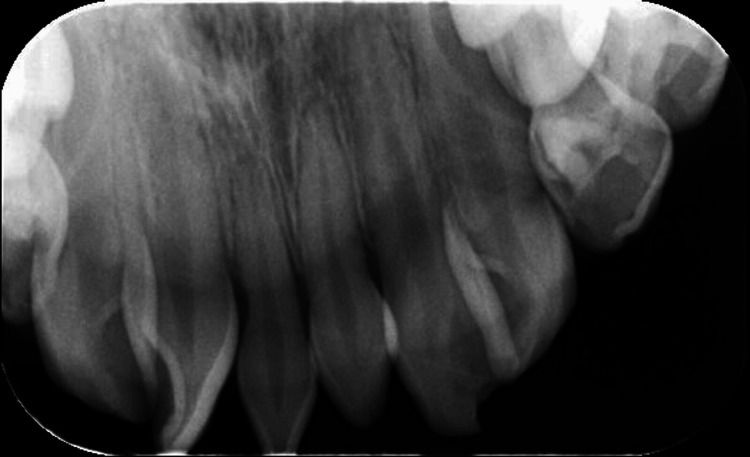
Periapical radiograph of the anterior maxillary region
